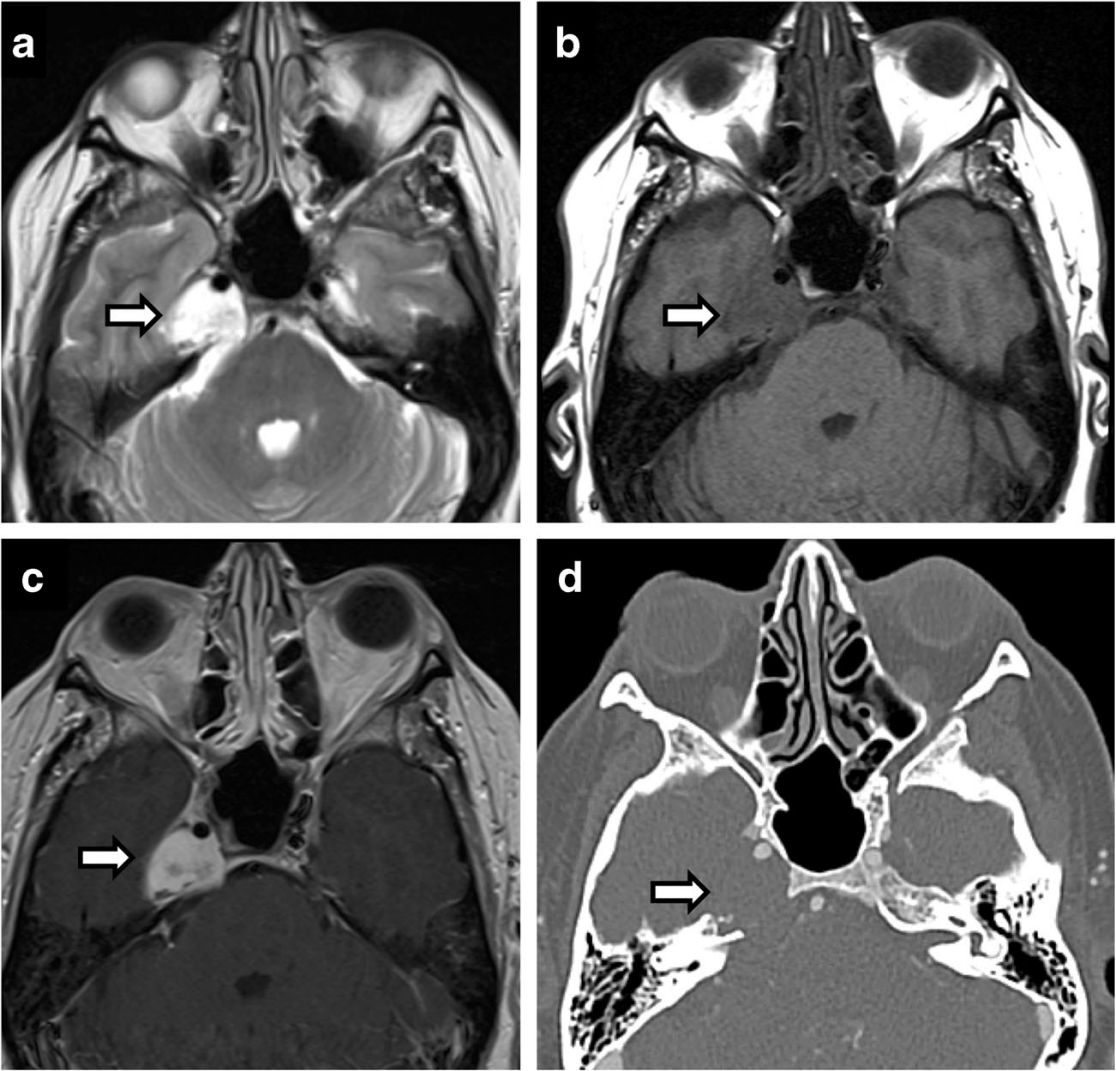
Chondrosarcoma. Axial T2 (a), axial T1 (b), axial T1 C+ (c), CT (d). A 61-year-old woman with effacement of the right Meckel’s cave by an expansile petrous apex mass that is hyperintense on T2, hypointense on T1 and shows avid enhancement on post contrast image. CT shows features of slow growing lesion. Note preserved Meckel’s cave on the left
